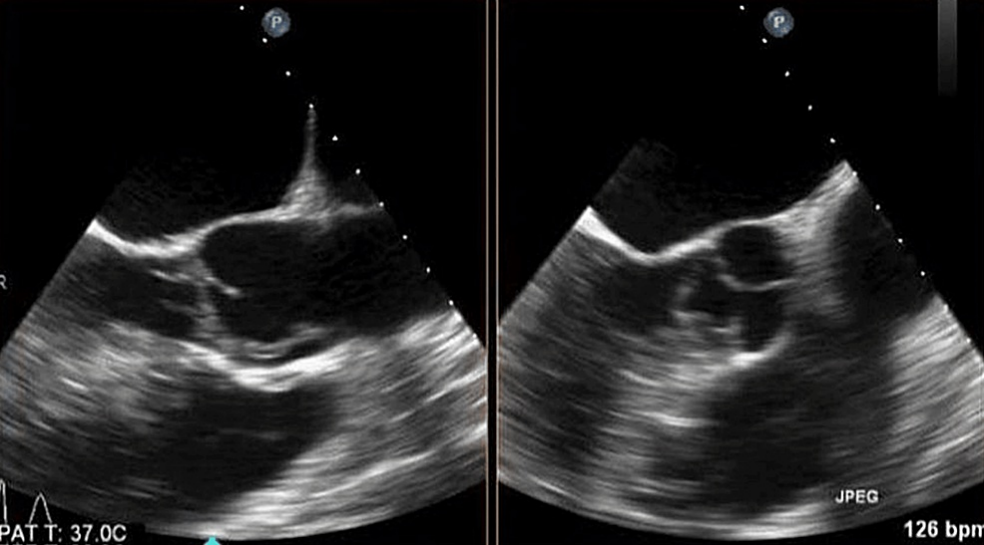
Transesophageal Echocardiogram showing Aortic Root Abscess
